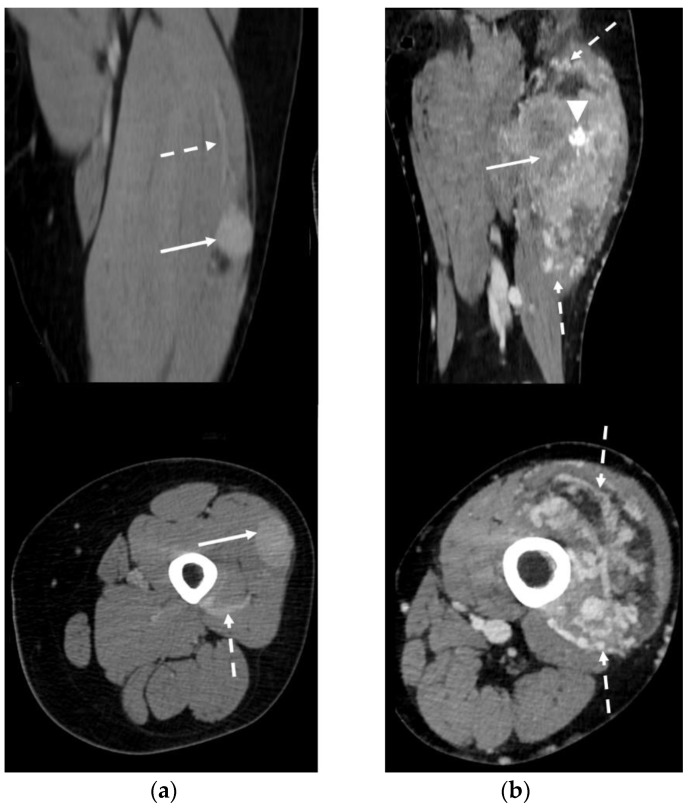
Contrast-enhanced CT in two patients affected by ASPS (arrows) of the thigh. Peri-/intratumoral feeding vessels can be clearly detected (dotted arrows). (a) Coronal and axial reconstructions demonstrate homogeneous lesion enhancement post contrast medium administration. (b) Coronal and axial reconstructions show a highly inhomogeneous enhancement pattern. Gross intratumoral calcifications can be noted (arrowhead).
